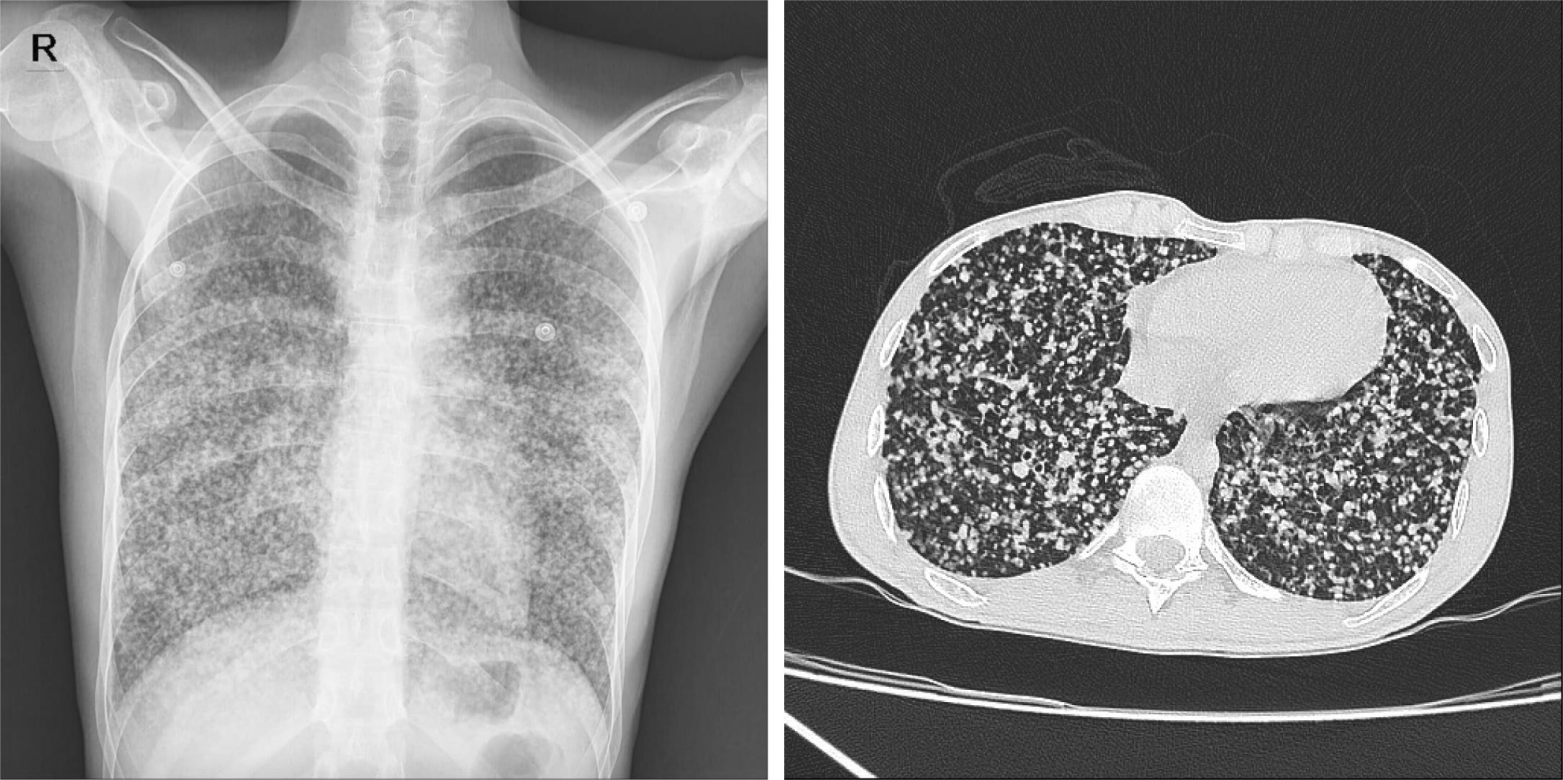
Patient's lung computed tomography.
